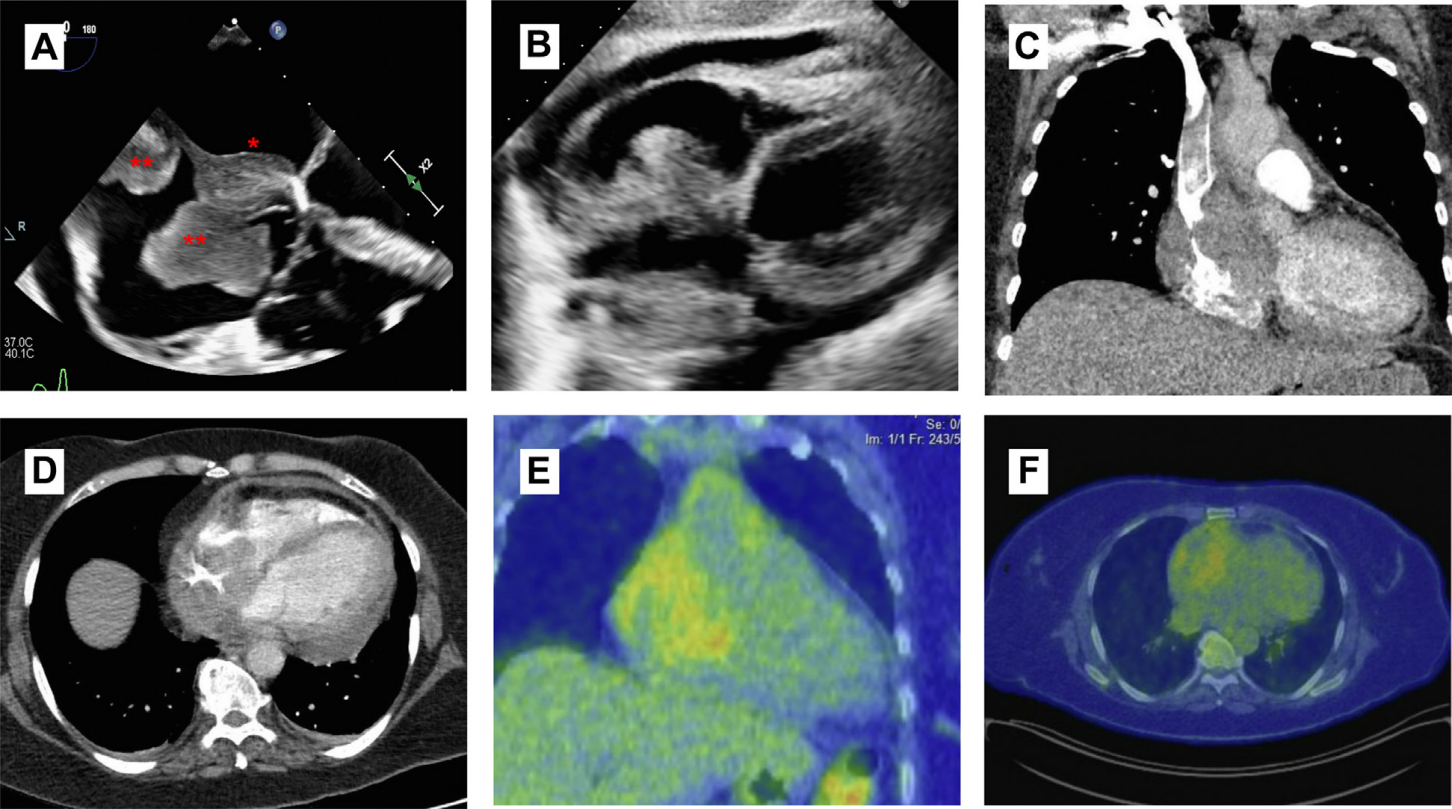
Imaging Results Right atrial mass demonstrated on multiple imaging modalities including echocardiography (A and B), computed tomography (CT) (C and D), and positron emission tomography (E and F). On transesophageal echocardiography (B), the multifocal mass is demonstrated (∗∗) along with a component crossing the interatrial septum (∗). The patient was ultimately diagnosed with a diffuse large B-cell lymphoma after a CT-guided biopsy of the tumor and appropriately initiated on chemotherapy with R-CHOP (rituximab, cyclophosphamide, doxorubicin, vincristine, and prednisone).
